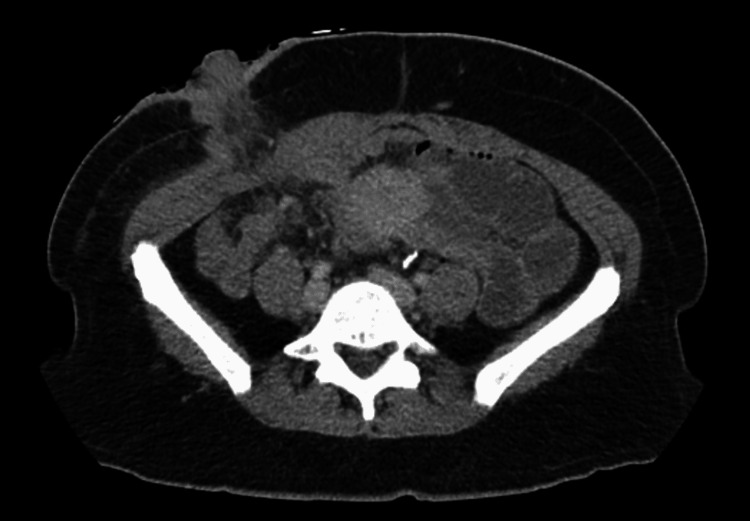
CT of the abdomen with contrast post-robotic total proctocolectomy with diverting loop ileostomy
